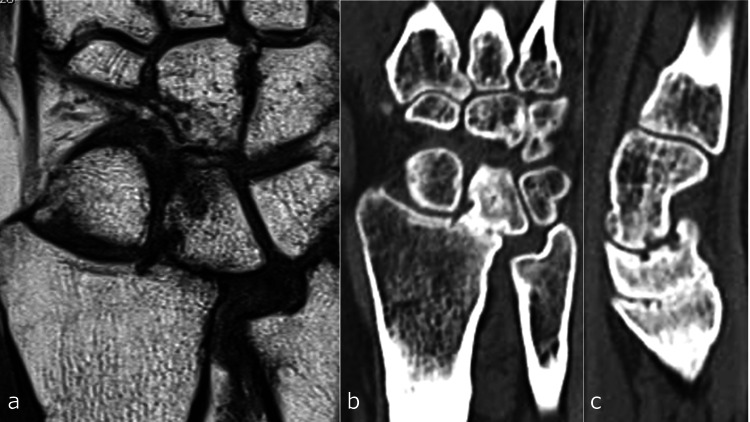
Imaging two years post-injury. a: Magnetic resonance imaging coronal view. b: Computed tomography (CT) coronal view. c: CT sagittal view.
